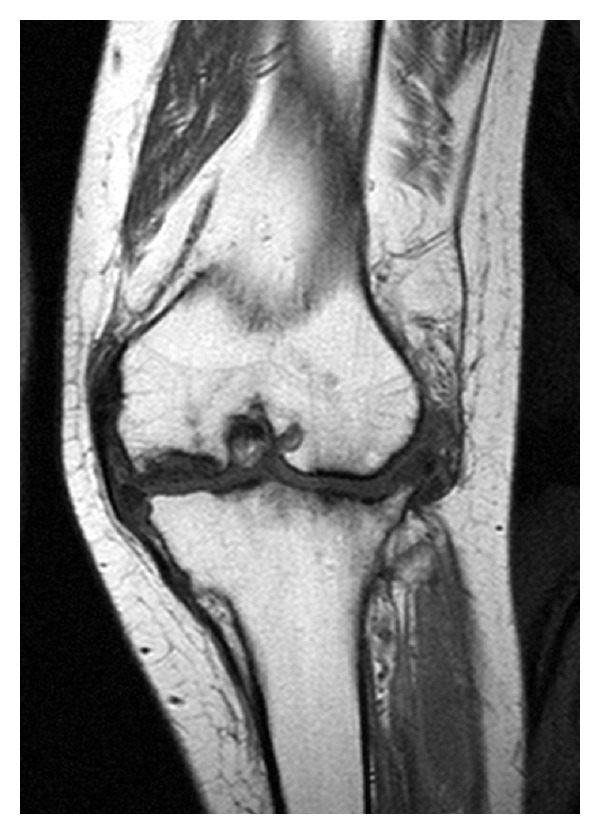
MRI T1 coronal view of the left knee.
